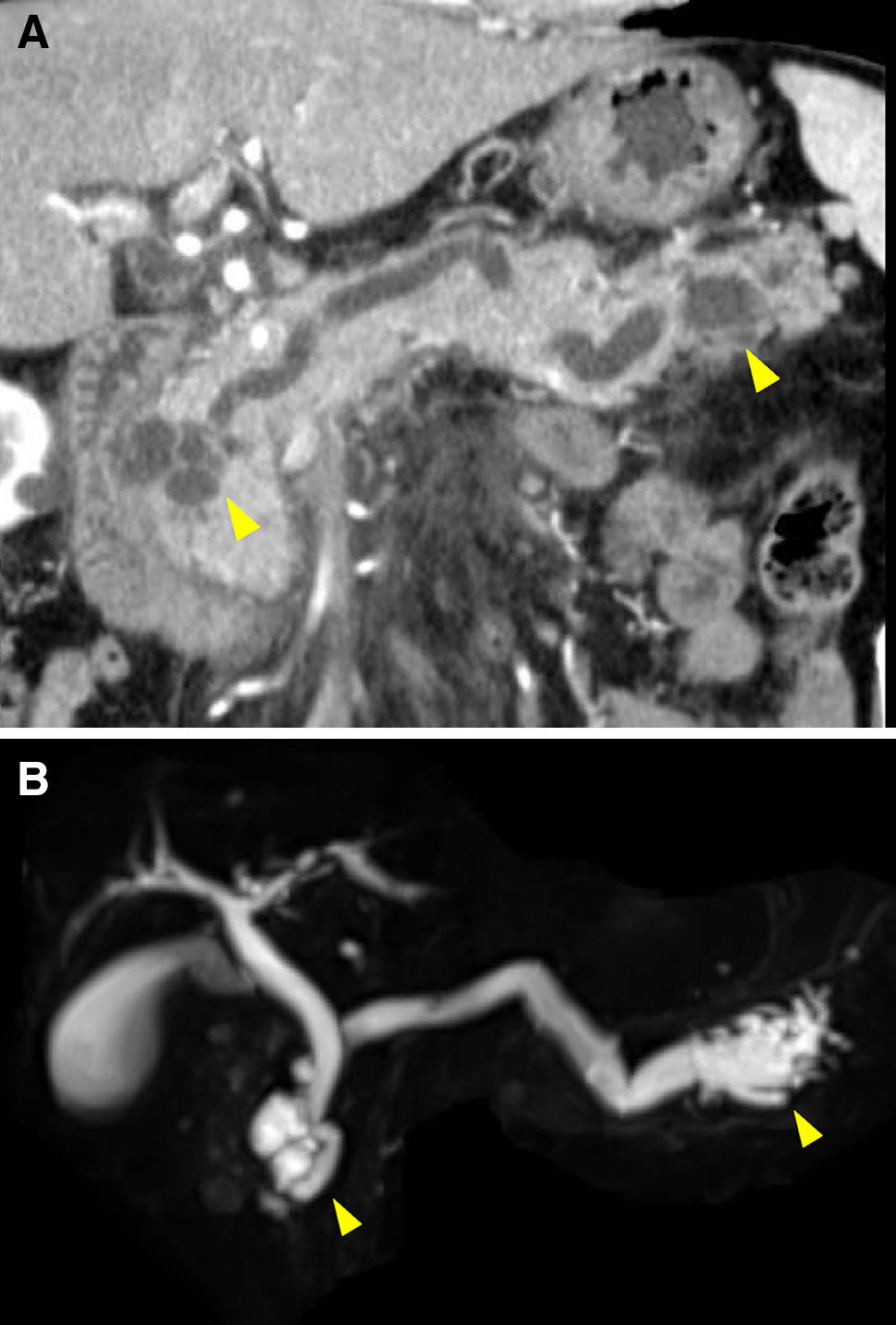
Abdominal CT (a) and MRCP (b) showed diffuse dilation of the main pancreatic duct, multiple cystic lesions in the pancreatic head, and obvious dilation of the main pancreatic duct in the pancreatic tail
